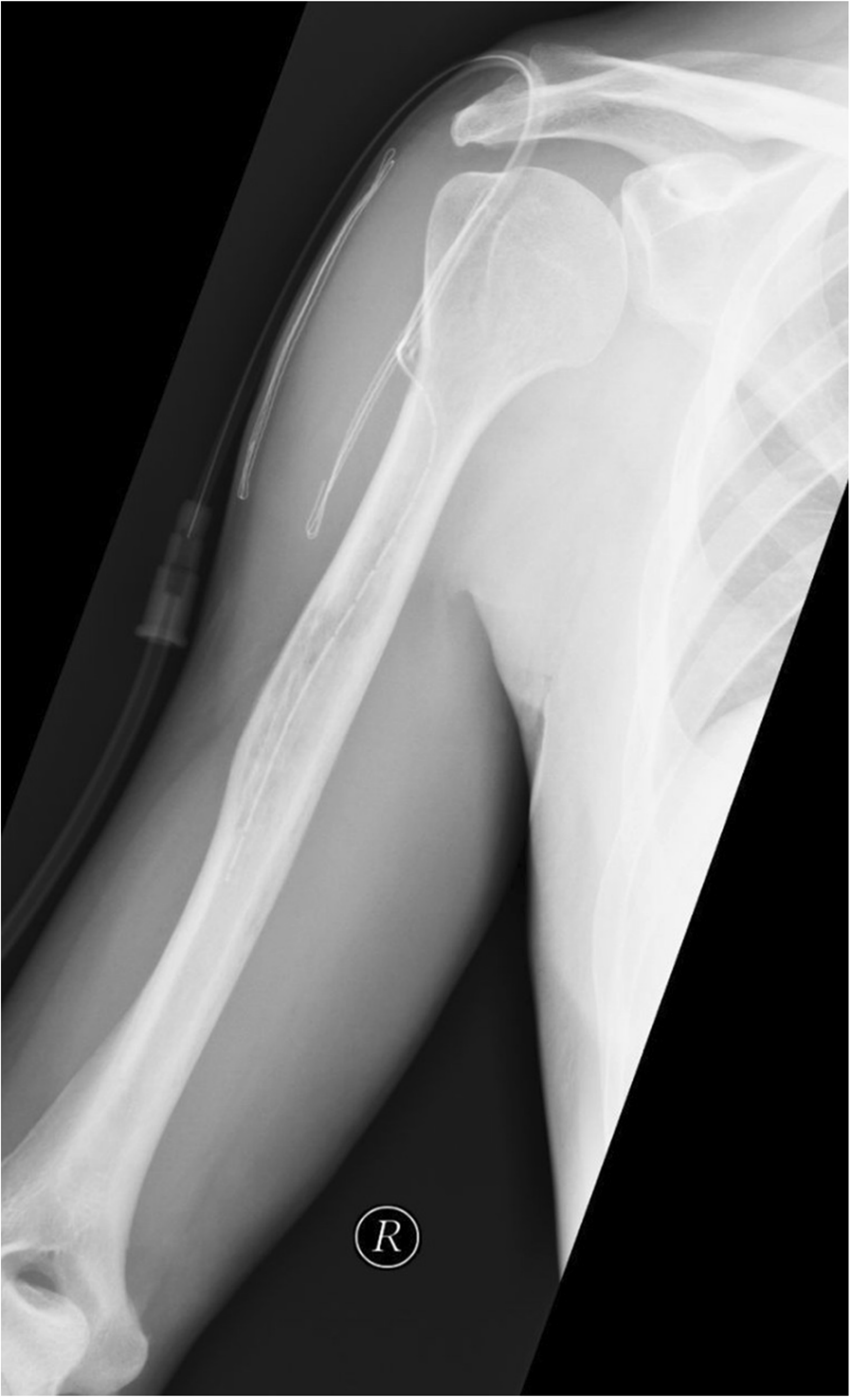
Radiograph of the right humerus, anterioposterior direction, postoperative status: drainage inserted via osseal window.
